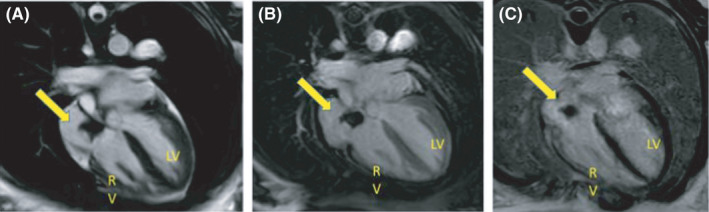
Cardiac MRI four chamber planes A, precontract 4 CH SSFP CINE still image showing a right atrial hypointense lobulated structure (yellow arrow), and post gadolinium early B, and delayed enhancement C, 4 CH views showing no contrast uptake within the right atrial structure, consistent with atrial thrombus
